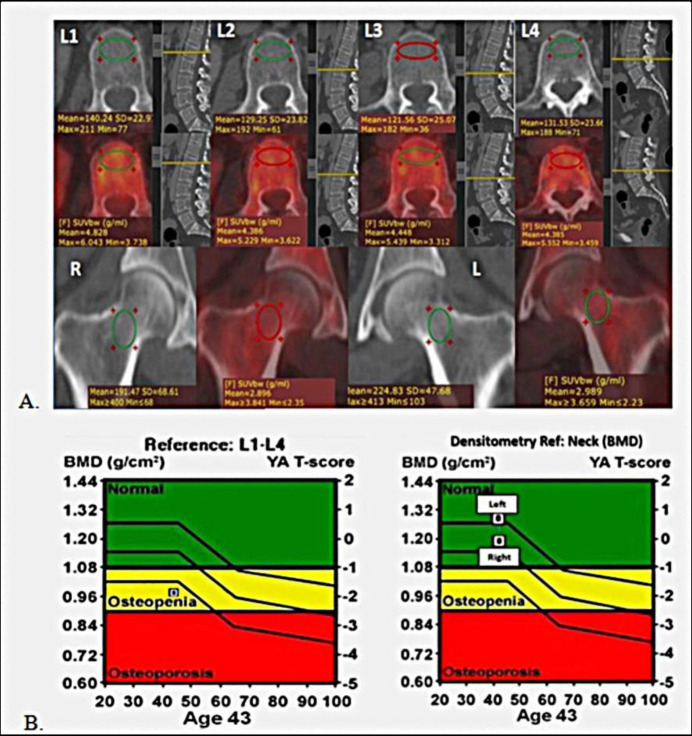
A 43 year old breast carcinoma patient, follow up PET/CT was done 7 months after Chemo ± hormonal therapy. Axial CECT (top row) shows significantly reduced L1-L4 HU (Mean CT attenuation ~130.6HU),whereas FNHU values were within normal range (mean FNHU ~208.1HU). Respective mean DXA T-scores were -1.9 and -0.2 for L1-L4 and femoral necks. Pre chemo ± hormonal therapy DXA was not done. This case also demonstrates that there can discrepancy between vertebral and femoral neck BMD and hence both should be screened on routine PET/CT scans in breast carcinoma patients
